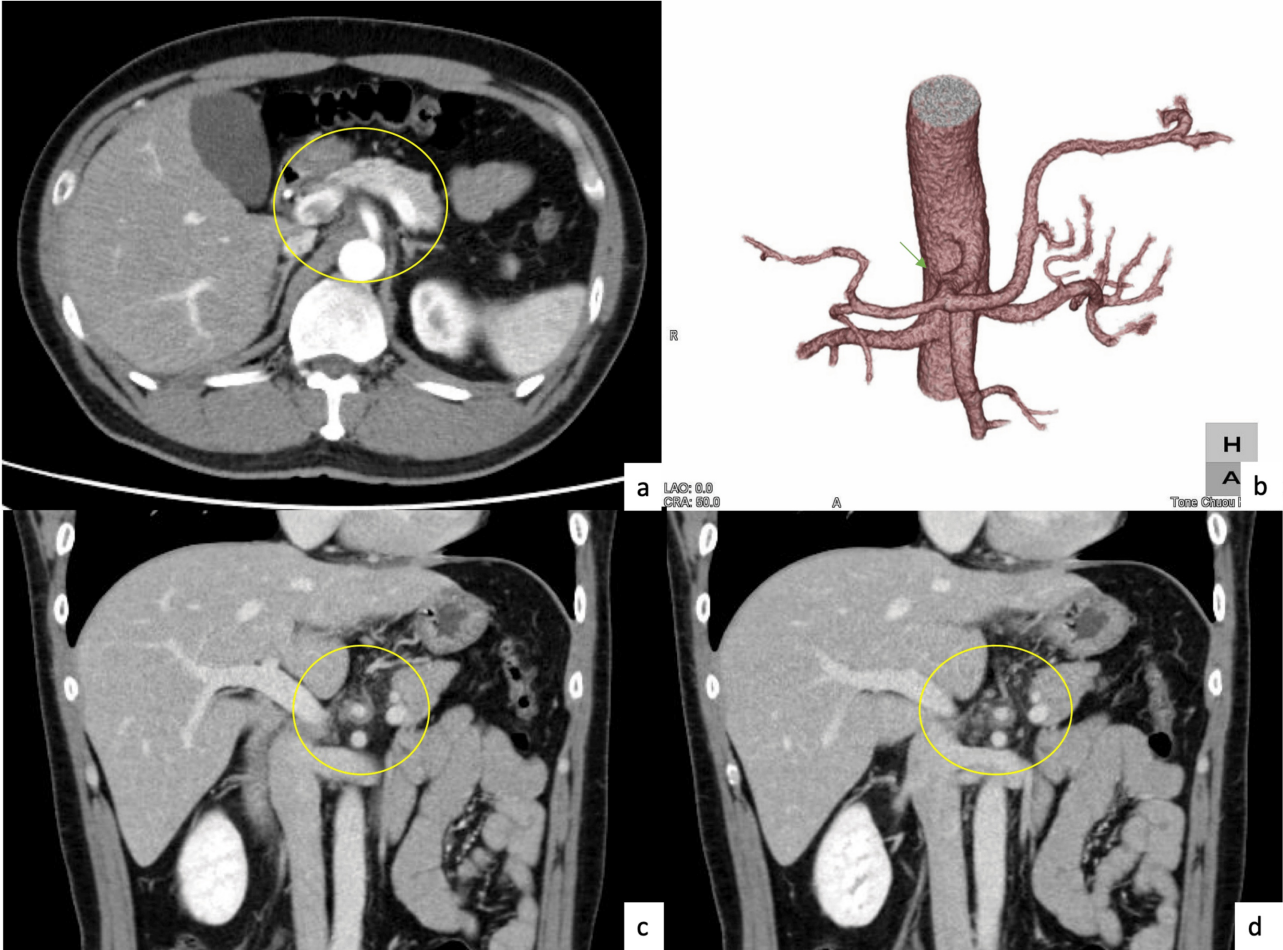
Abdominal CE-CT images False lumen occlusion-type celiac artery dissection (encircled) CE-CT: Contrast-enhanced CT
